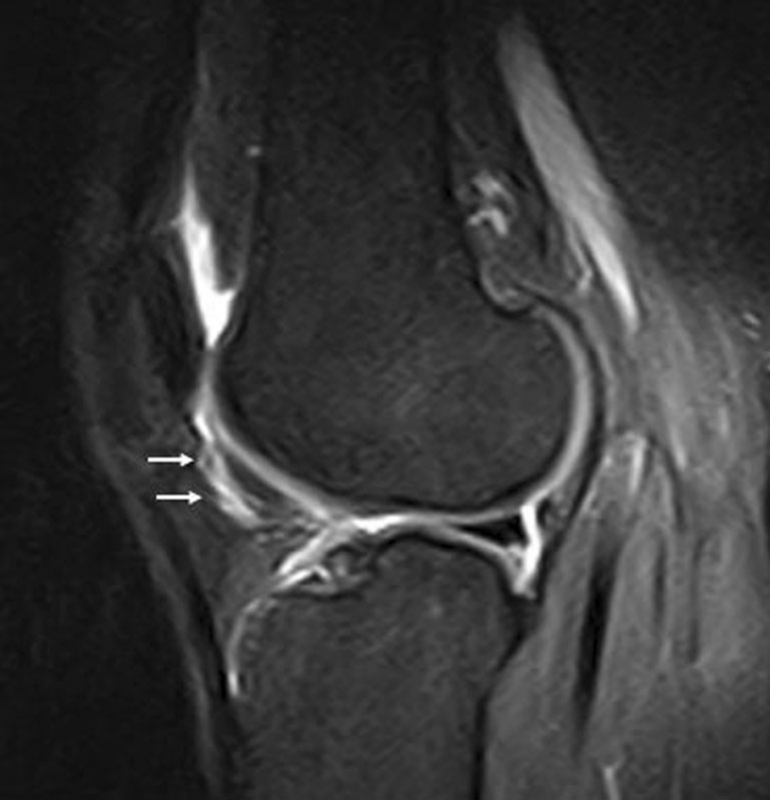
HFP acute trauma. MRI proton density with fat saturation image, after direct trauma, shows HFP fragmentation (*arrows*)
